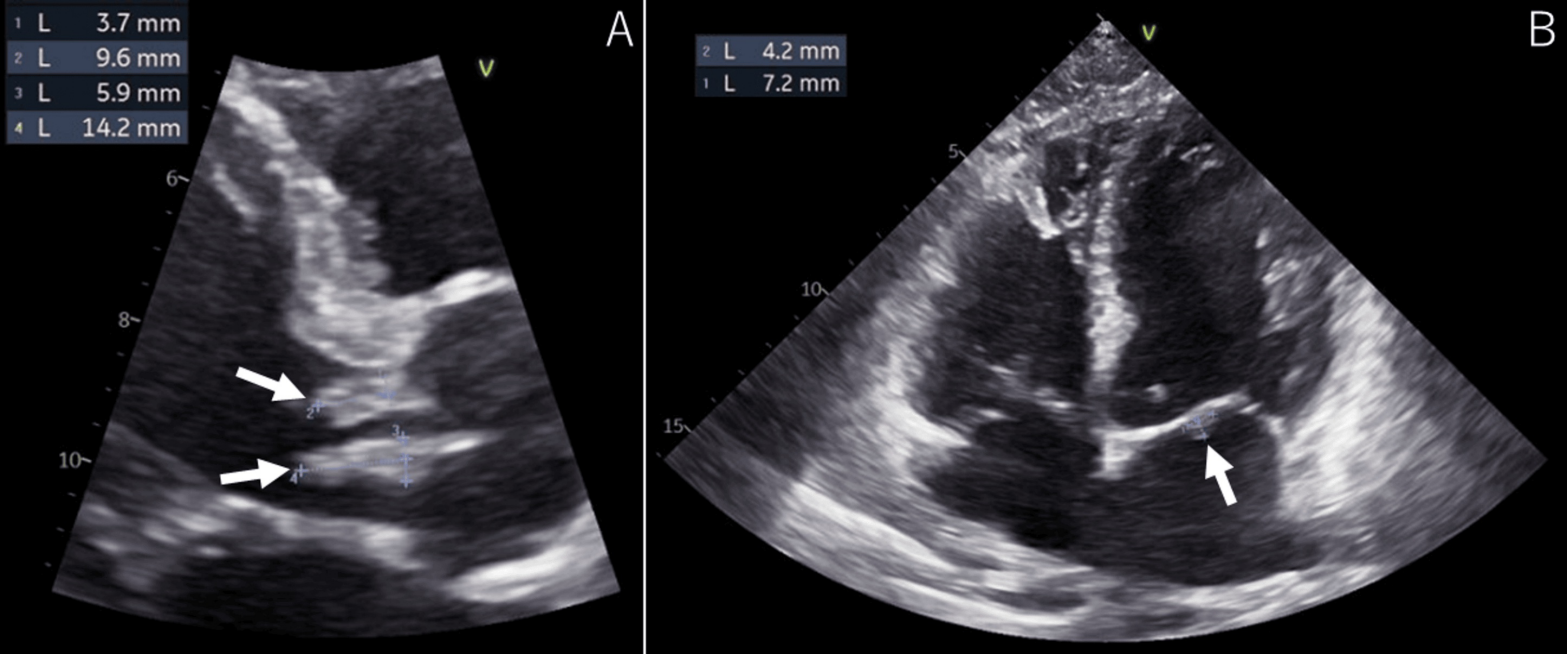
Echocardiography. (A) A club-shaped mass is observed on the aortic and mitral valves (arrow).
